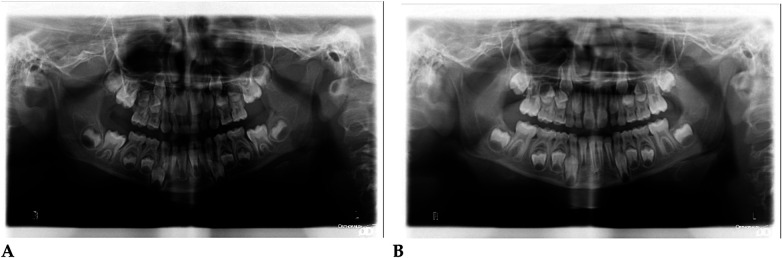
Pre- (A) and post-treatment (B) panoramic radiographs of case 10.
